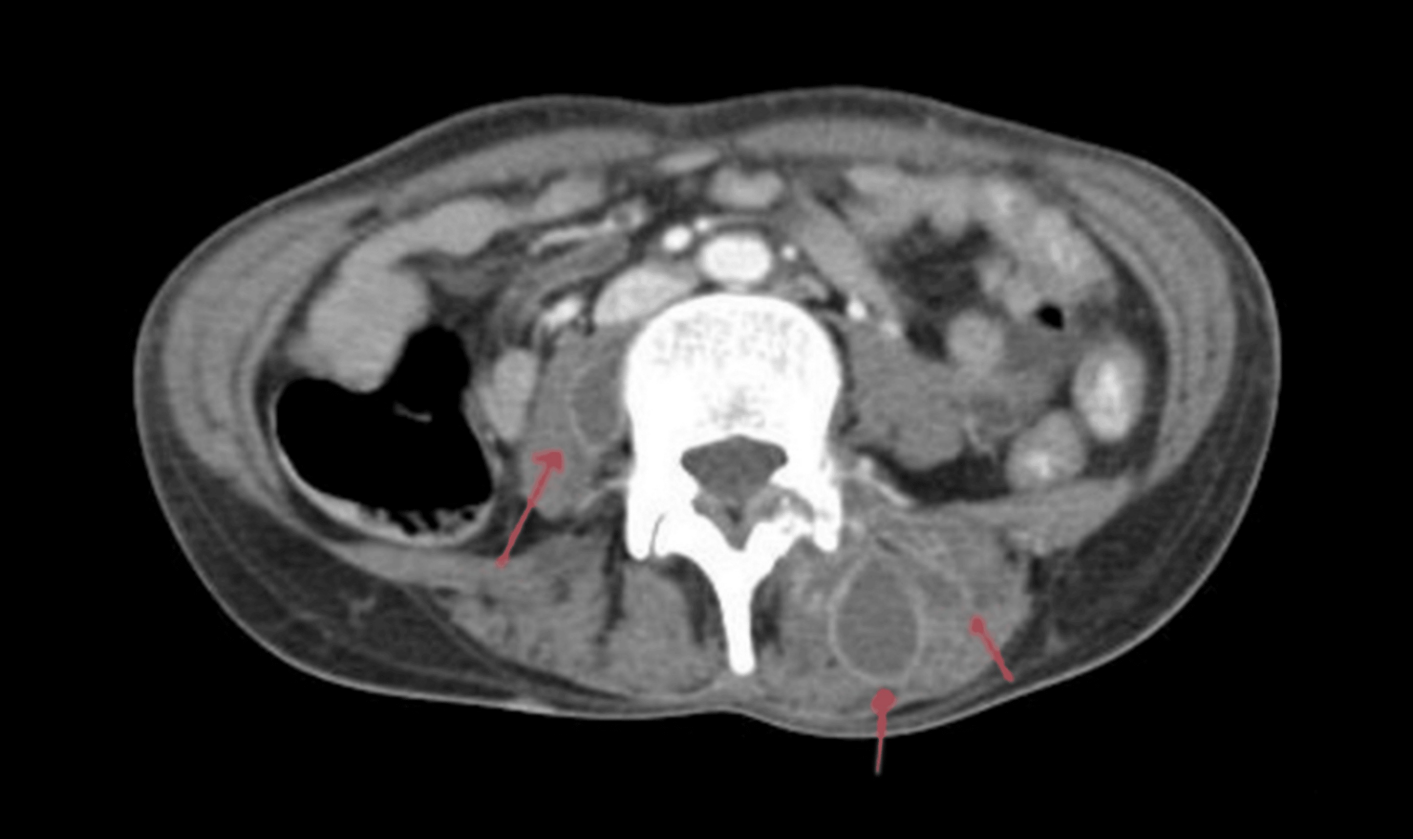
CT abdomen showing multiple abscesses within the right psoas muscle and left paraspinal muscles.
